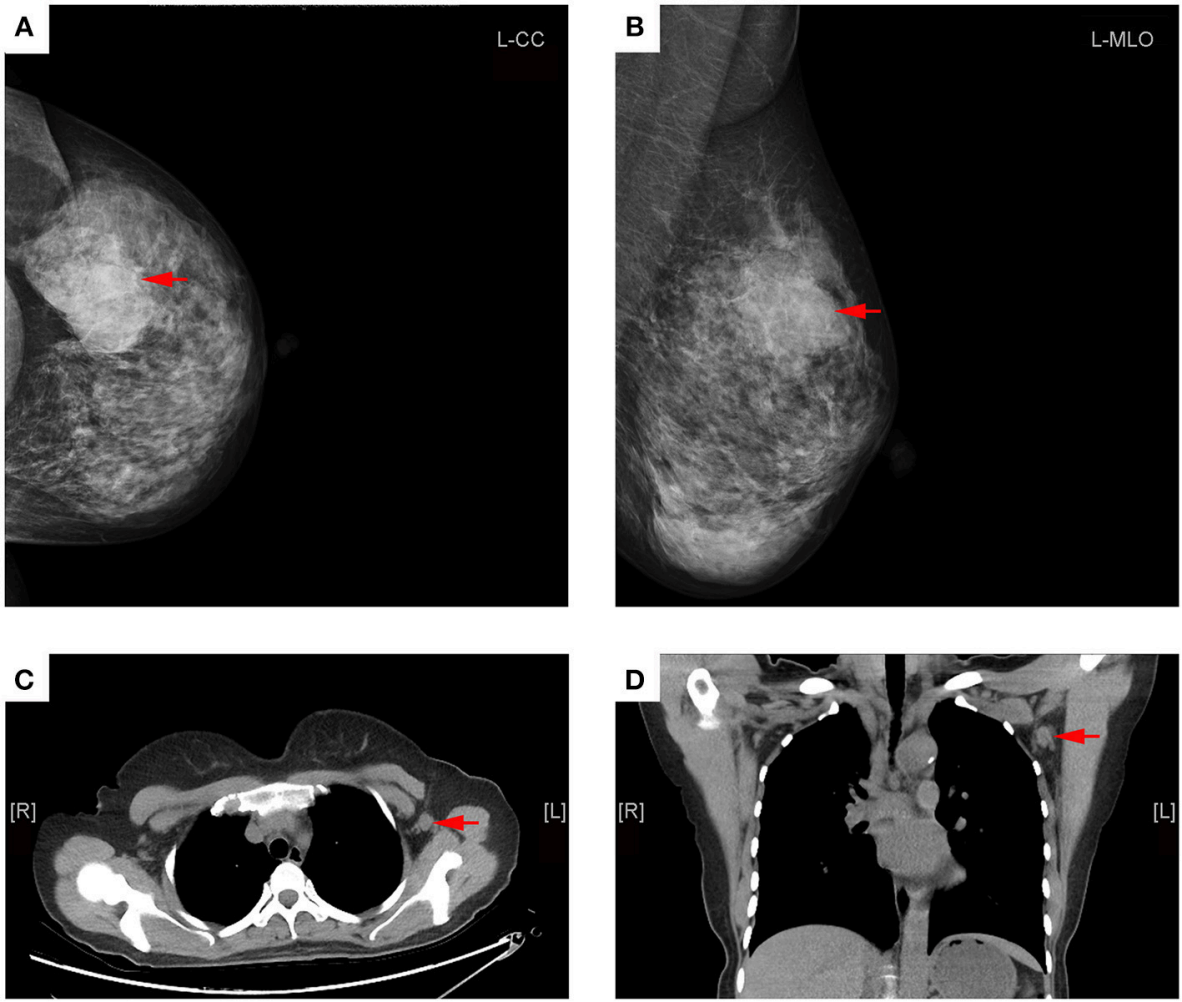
Representative mammography and MDCT images of a 45-year-old woman with left primary breast cancer (stage pT2N1). (A) Mammography of craniocaudal image showed a mass in the lateral of the left breast, lobulated, and slightly high-density shadows, about 56 × 36 mm in size, with rough edges and part of the edge was fuzzy (red arrows). (B) Mammography of mediolateral oblique images showed a mass in the upper quadrant of the left breast, lobulated, and slightly high-density shadows (red arrows). (C) Transverse CT image showed an axillary lymph node with round shape and non-fatty hilum, a cortical thickness about 11 mm (red arrow). (D) Coronal CT image showed this lymph node with round shape and no fatty hilum, a long-axis diameter of about 15 mm, and a short-axis diameter of about 13 mm (red arrows). The patient underwent axillary lymph node dissection, 15 lymph nodes were removed, and two lymph nodes were proved to be pathologic positive.
